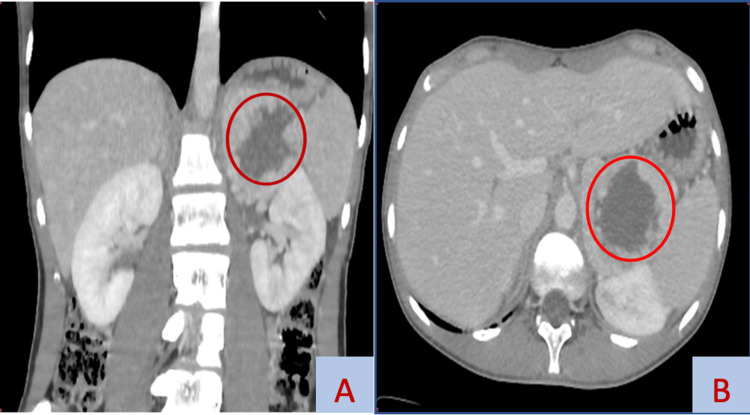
Frontal (A) and transverse (B) abdominal CT sections showing a 60 x 72 x 75 mm tissue mass between the spleen and peritoneum with a necrotic appearance (red circle).
